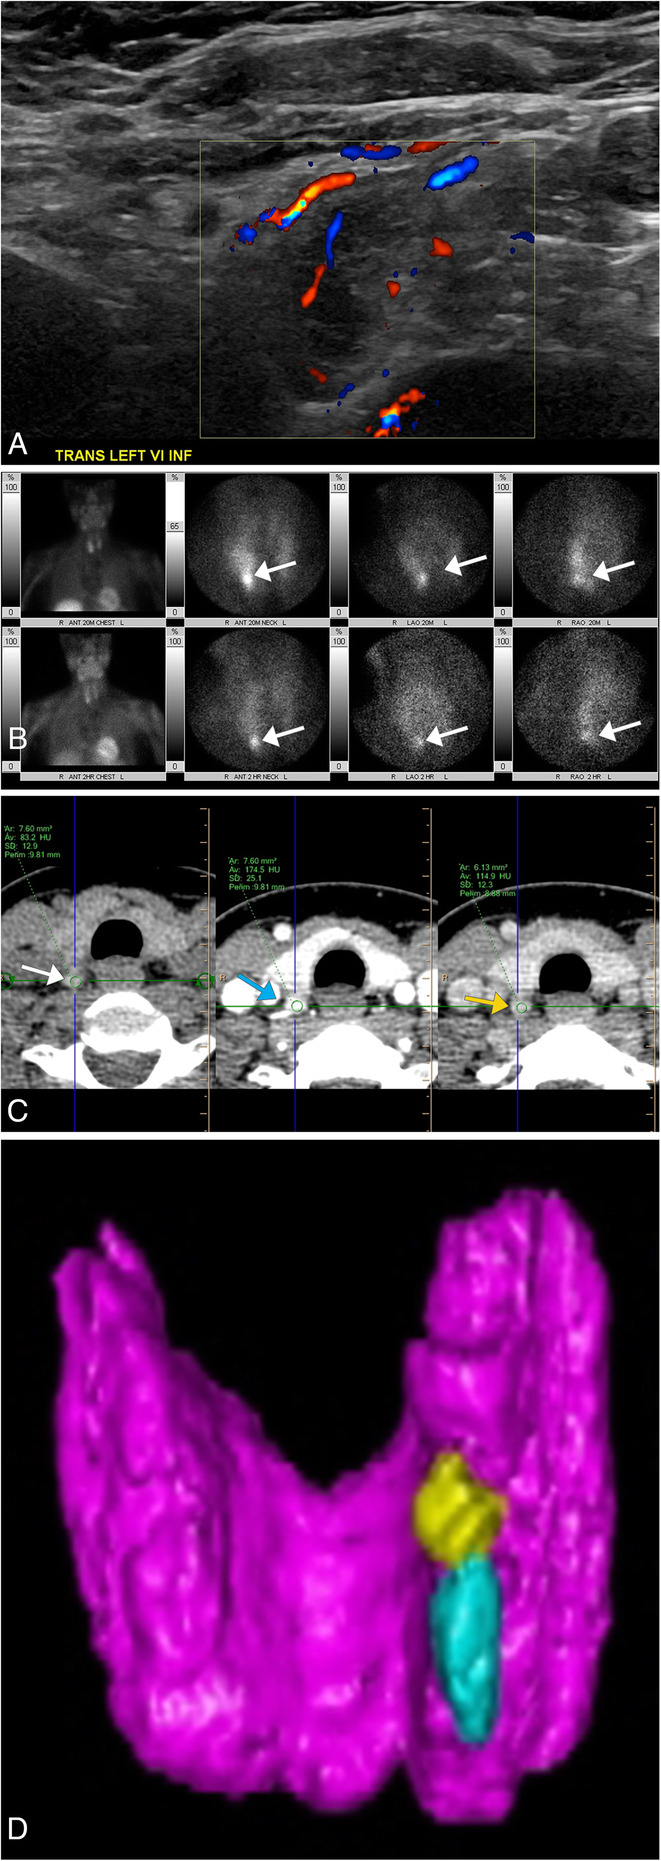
Parathyroid Adenoma. a Grayscale with Doppler ultrasound image demonstrates an ovoid well-circumscribed hypoechoic lesion containing cystic anechoic areas, located at the inferior posterior aspect of the left thyroid lobe. Doppler shows the vessels feeding the adenoma. b Tc99 sestamibi scan demonstrates increased radiotracer uptake in the lower pole of the right thyroid lobe in a companion case with persistent uptake 2 h later (arrows). Multiphasic 4-dimensional CT imaging of the parathyroid glands was performed in the same patient as the nuclear medicine study. c Axial precontrast (left), arterial phase (middle), and late arterial/delayed phase (right) images identified two enhancing nodules in the posterior aspect of the right lower thyroid lobe which washed out on delayed phase imaging (upper nodule shown). This nodule measured 83 HU precontrast (white arrow), 175 HU on arterial phase (blue arrow), and 115 HU on delayed phase (orange arrow). d 3D reconstructions created from the CT imaging demonstrate the position of the two nodules (yellow and turquoise) relative to the thyroid gland (purple) on the posterior view, assisting in surgical pre-procedure planning
